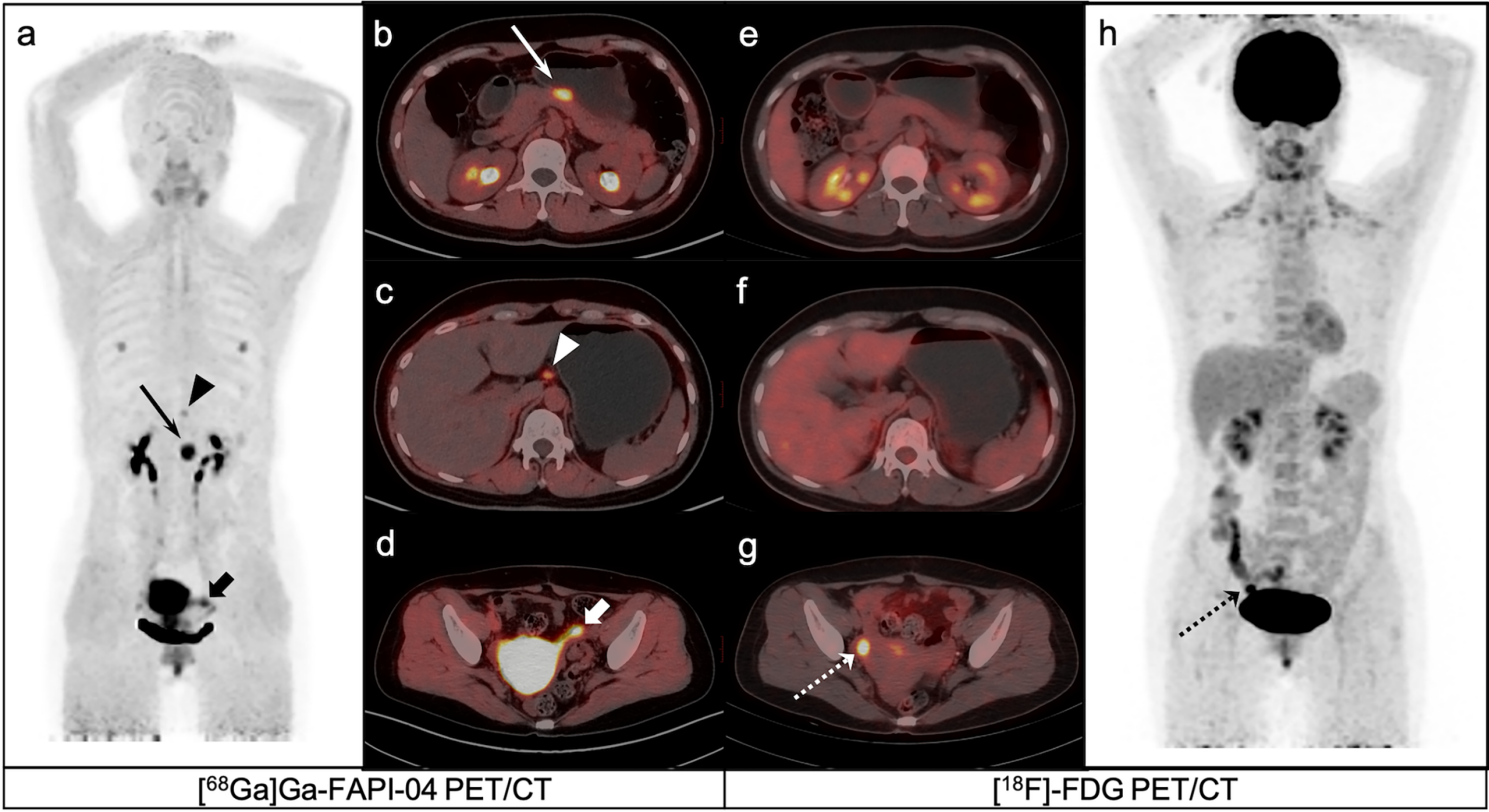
A 40-year-old female patient with poorly differentiated gastric adenocarcinoma based on pathological biopsy under gastroscopy. [^68^Ga]Ga-FAPI-04 PET/CT (A–C) revealed high uptake in the primary tumor (slender arrows, SUVmax=10.3) and lesser curved lymph nodes in the stomach (triangles, SUVmax=3.2), but negative uptake was observed on [^18^F]-FDG PET/CT (E, F, H). [^18^F]-FDG PET/CT (H) revealed brown fat visualization at the base of the neck and in the supraclavicular fossa. [^18^F]-FDG PET/CT (G) revealed high uptake in the right ovarian (dashed arrows, SUVmax=8.2), but negative uptake was observed on [^68^Ga]Ga-FAPI-04 PET/CT (D).
